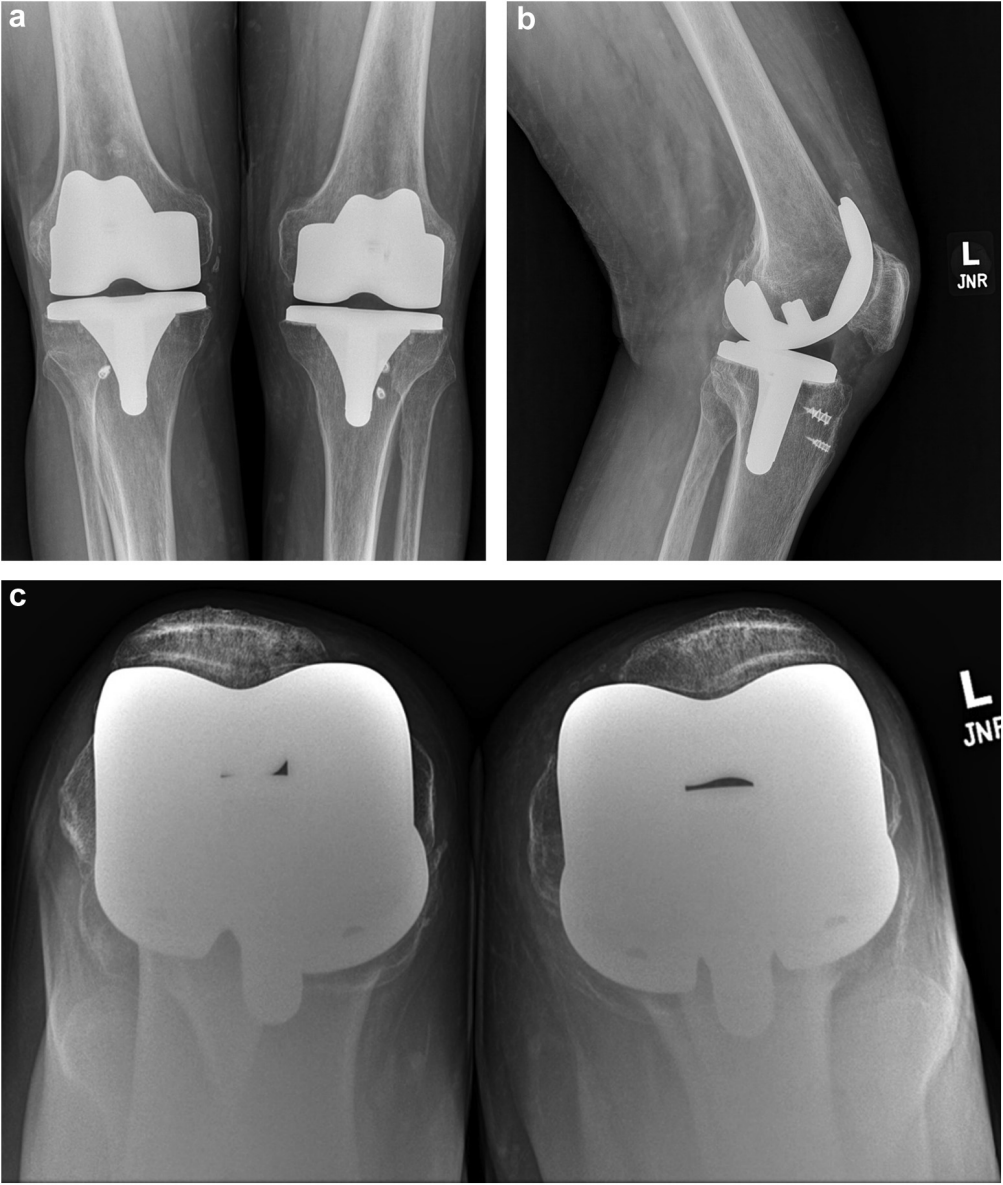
Anteroposterior (a), lateral (b), and sunrise (c) radiographs demonstrating a well-aligned left TKA after successful completion of a conservative treatment regimen for lateral tibiofemoral dislocation with MCL disruption.
